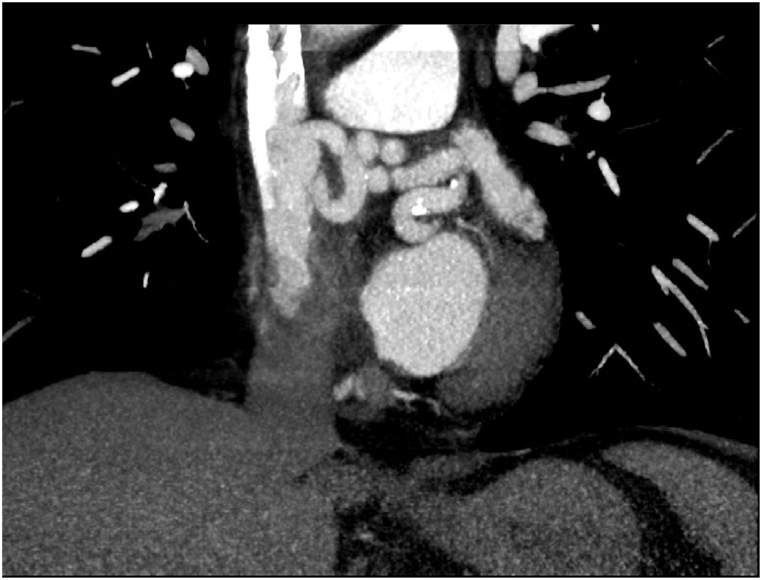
Sagittal section CT with sinuous fistula circumflex artery and superior vena cava.
